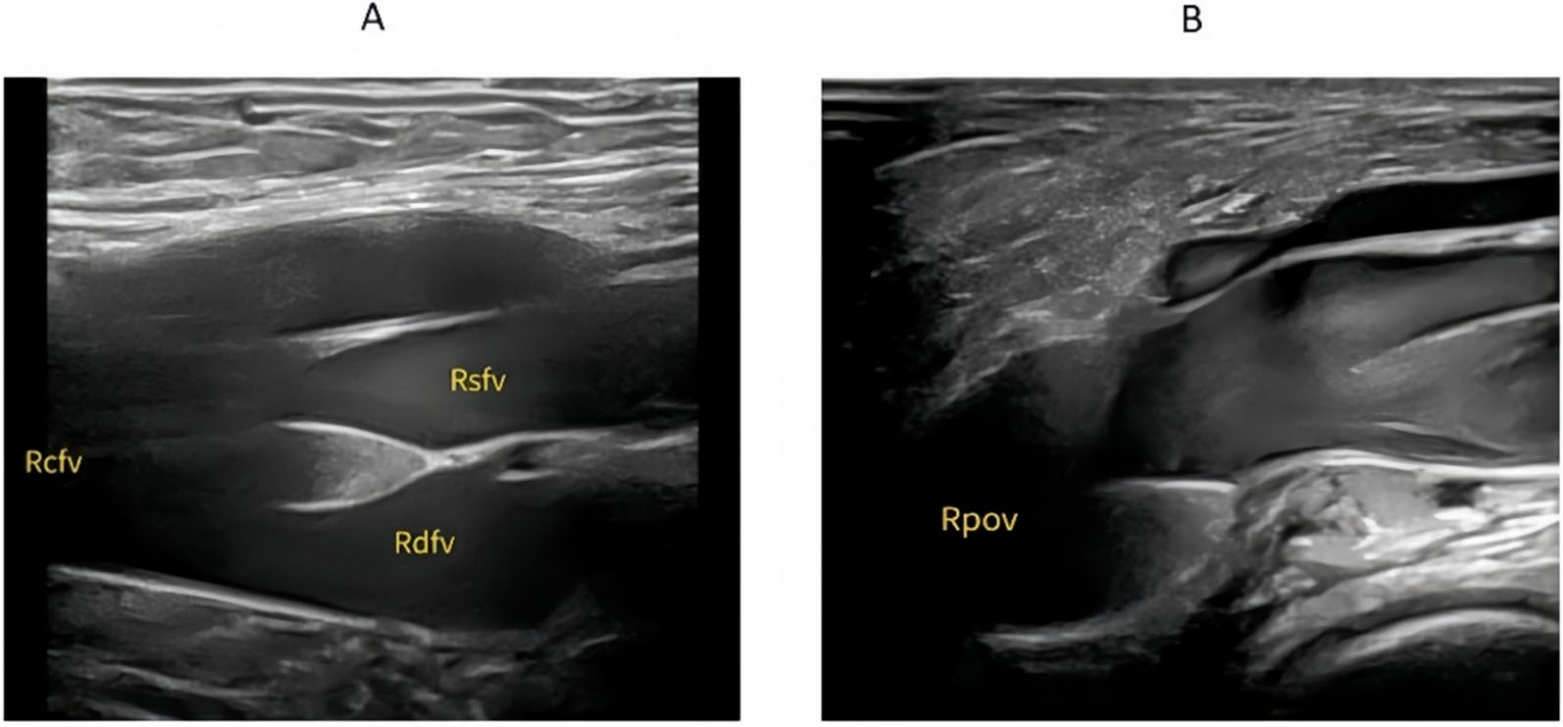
Valve-associated “blizzard” signs in lower extremity veins. Representative Doppler ultrasound images showing “blizzard” signs localized in the femoral and popliteal veins. Rcfv: right common femoral vein; Rsfv: right superficial femoral vein; Rdfv: right deep femoral vein; Rpov: right popliteal vein
